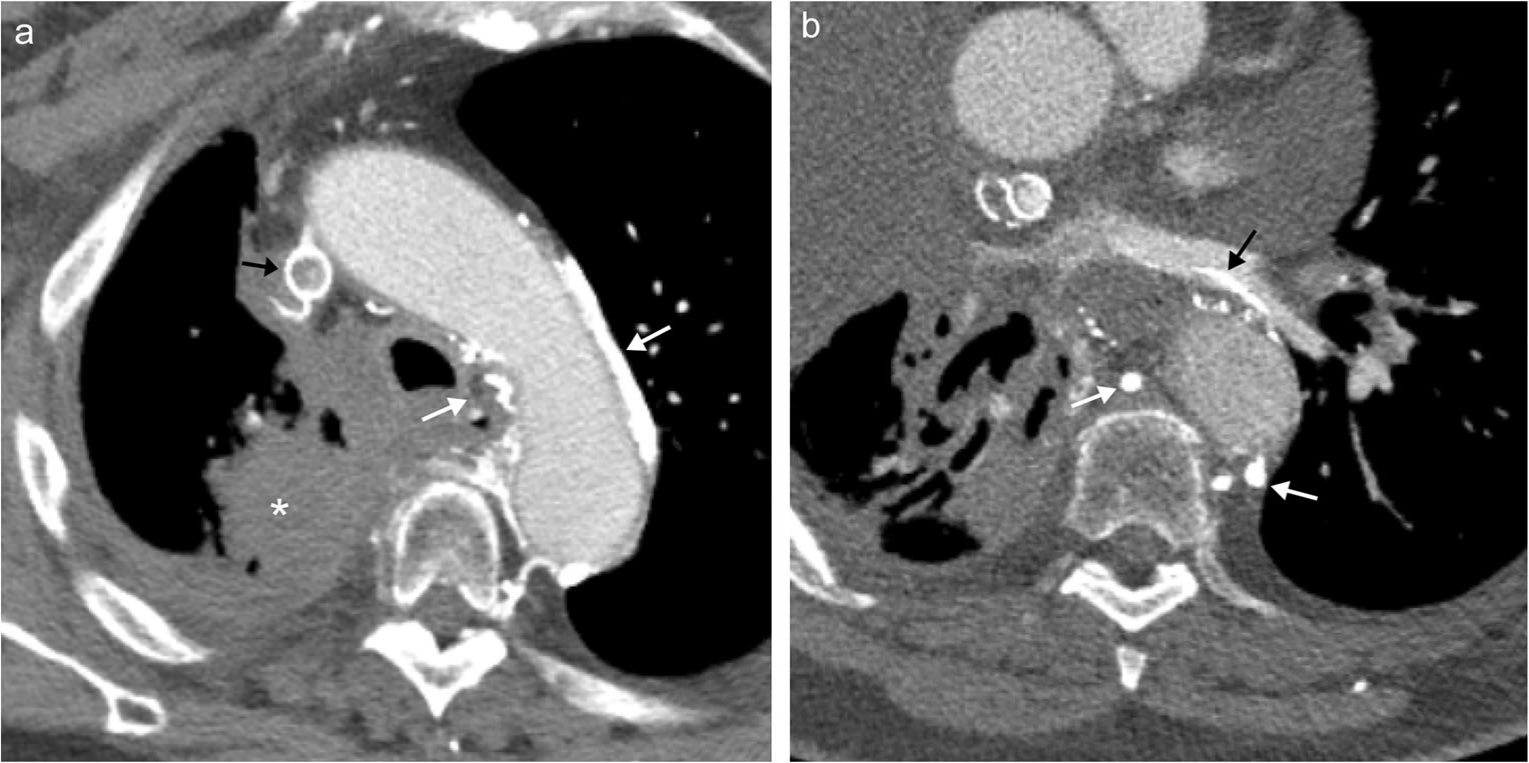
Pulmonary venous collaterals. a Axial contrast-enhanced CT image demonstrates upper right lobe squamous cell carcinoma (*) with metastatic disease obstructing the superior vena cava and stent (*black arrow*). Note extensive collateral formation (*white arrows*). b Axial CT image showing high-density contrast flow into the pulmonary venous system (*black arrow*) secondary to collateral formation (*white arrows*) with a right-to-left shunt in the setting of superior vena cava obstruction
